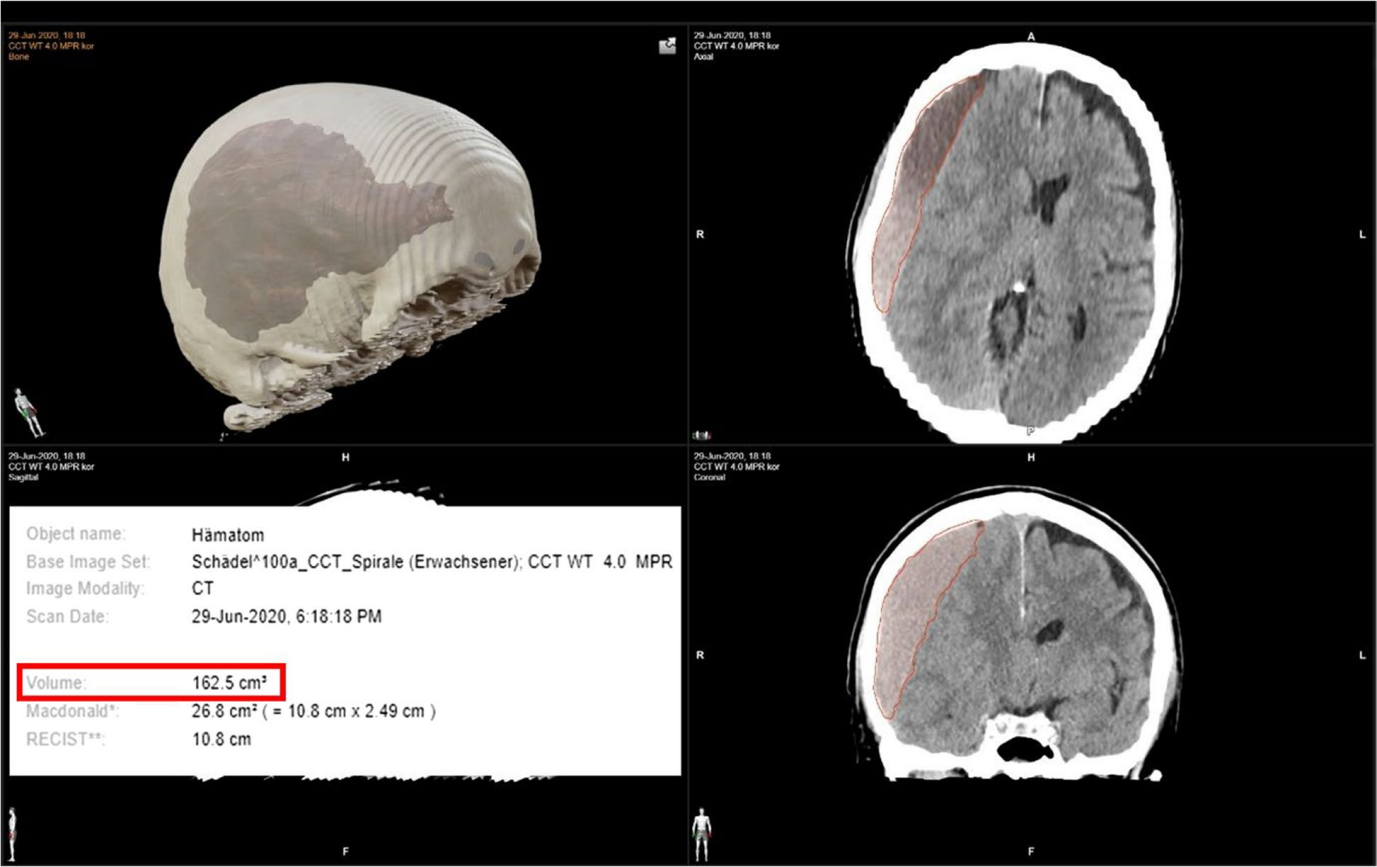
Preoperative volume measurement of a right-sided hemispheric chronic subdural hematoma using the CT data set
